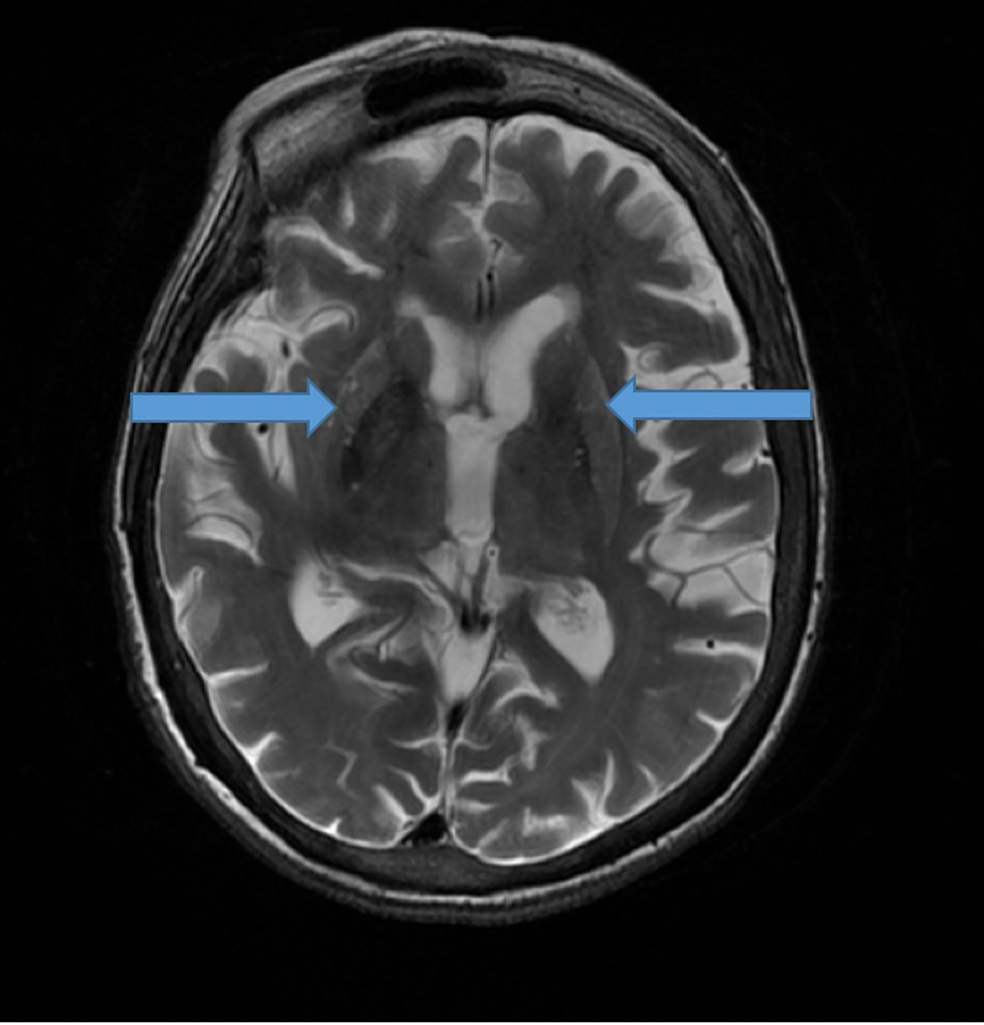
T2-weighted magnetic resonance image showing bilateral symmetric signal abnormality involving the caudate and putamen (blue arrows).
